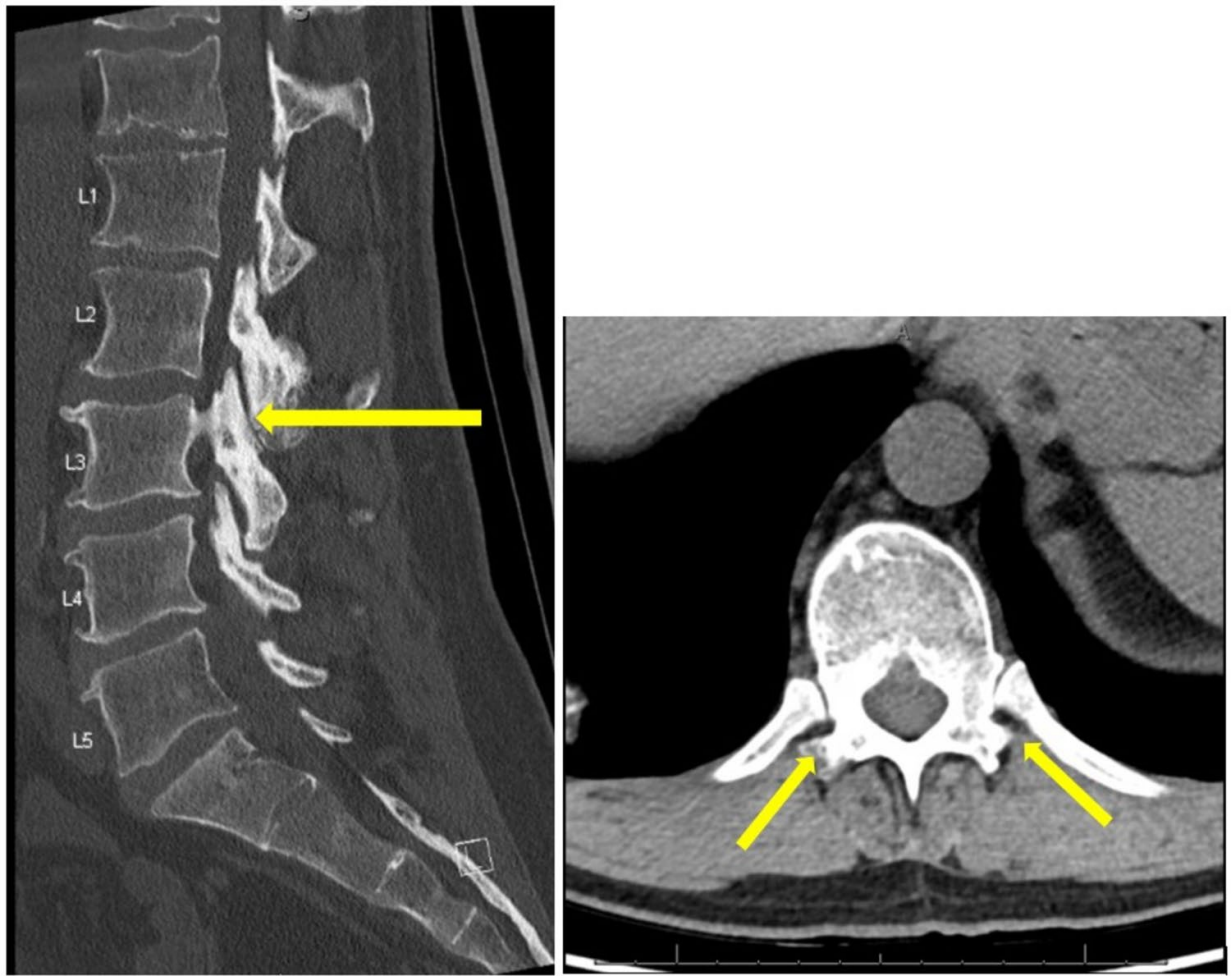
Low-dose computed tomography (CT) scan of the lumbar spine demonstrating bilateral pars at L3 with surrounding sclerosis (yellow arrows)
